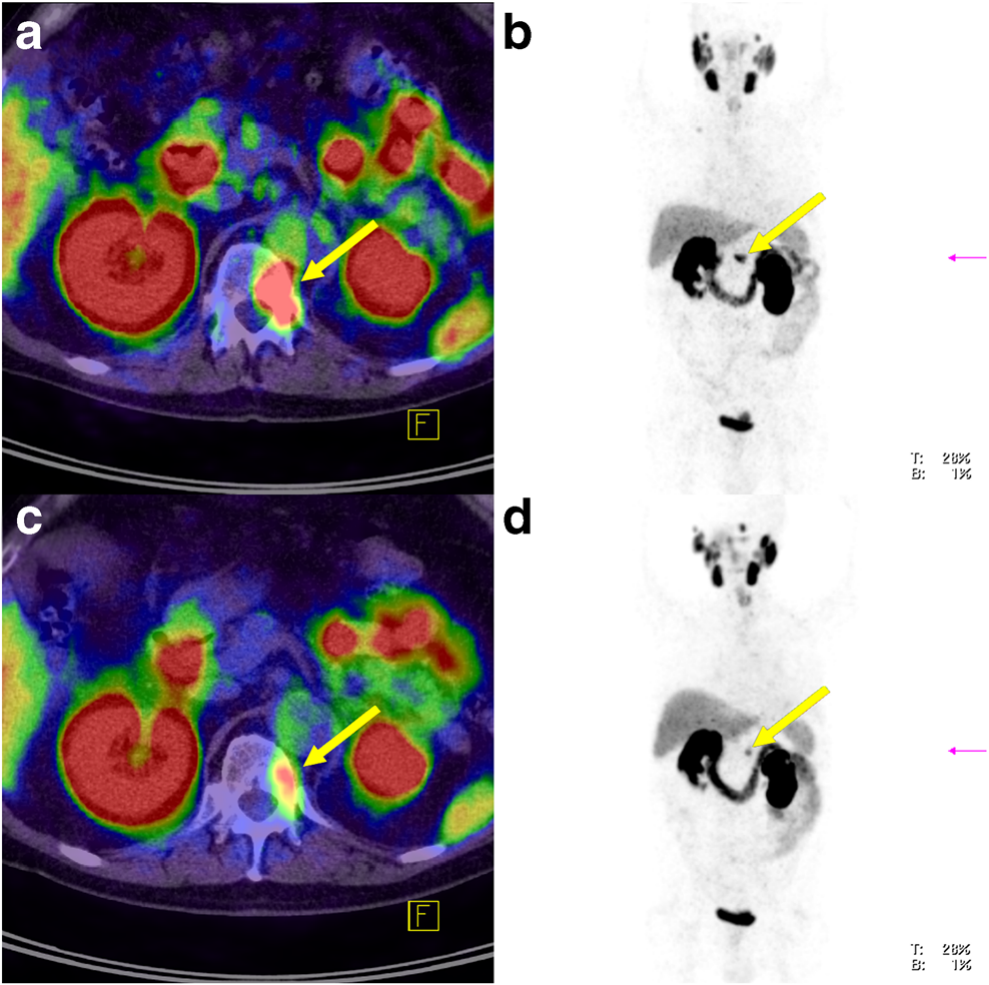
Bone metastasis in an example patient (patient 1; *yellow arrows*) without ADT (a, b) and during ongoing ADT (c, d) 323 days after the first scan. a Fused PET and CT image without ADT. b Maximum intensity projection image of the PET data without ADT. c Fused PET and CT image during ongoing ADT. d Maximum intensity projection image of the PET data during ongoing ADT
